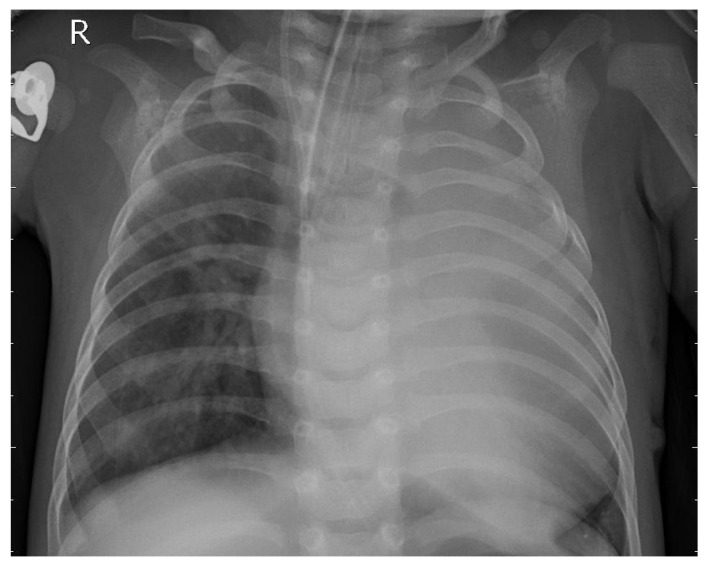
Bacterial pneumonia patient X-ray.
